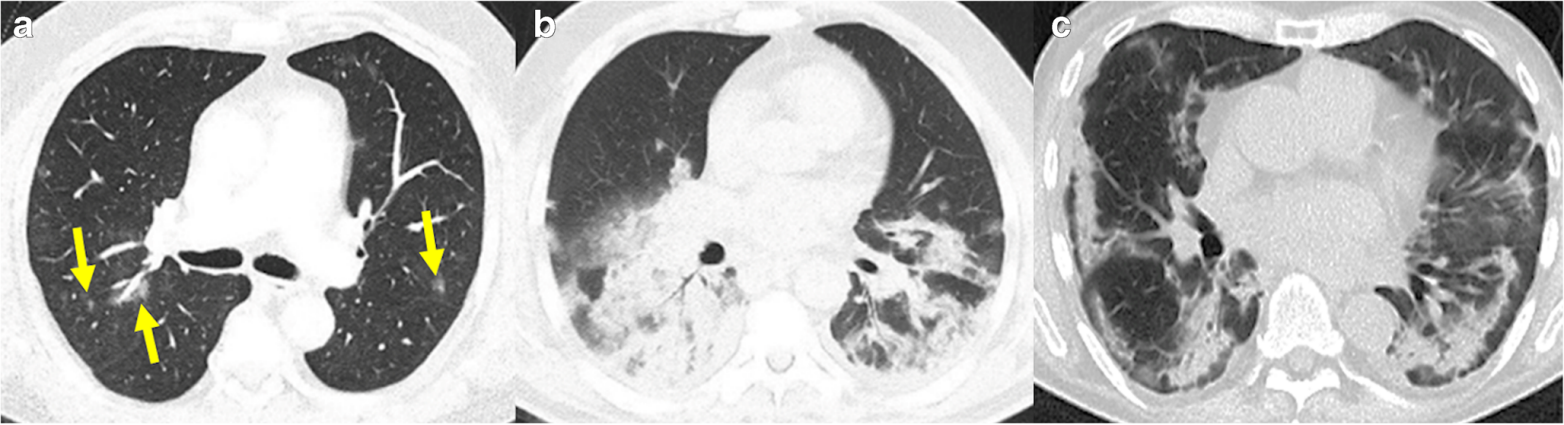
Three different patients with COVID-19 related abnormalities in the lungs shown by non-contrast CT. a A 55-year-old male patient with COVID-19 diagnosed 1 day before. CT showing ill-defined ground glass nodules in both lungs (yellow arrows). b A 49-year-old male patient with acute dyspnea for 4 days. CT showing extensive ill-defined consolidations with positive air-bronchograms in both lower lobes: COVID-19 was confirmed by RT-PCR. c A 60-year-old male patient with consolidations in the lung periphery sparing the subpleural space. The findings are compatible with organizing pneumonia indicating ongoing repair of the known COVID-19 pneumonia
